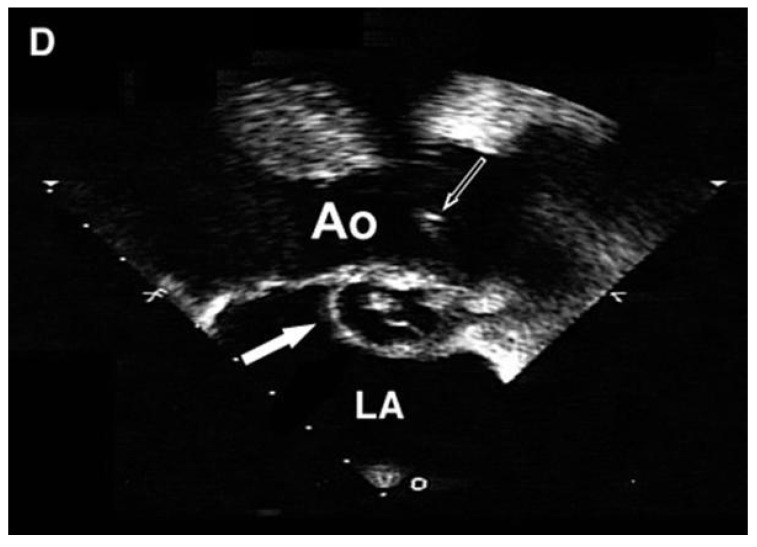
(A,B): Transthoracic parasternal and apical two chamber views showing the pseudoaneurysm and its proximity to the prosthetic aortic valve (see arrow). (C,D): Transesophageal echocardiography delineating the pseudoaneurysm and encroachment of the MAIVF (indicated by bold arrow). The aortic valve is indicated by the open arrow. MAIVF: mitral-aortic intervalvular fibrosa; LV: left ventricle; RV: right ventricle; LA: left atrium; RA: right atrium; Ao: aorta [43].
